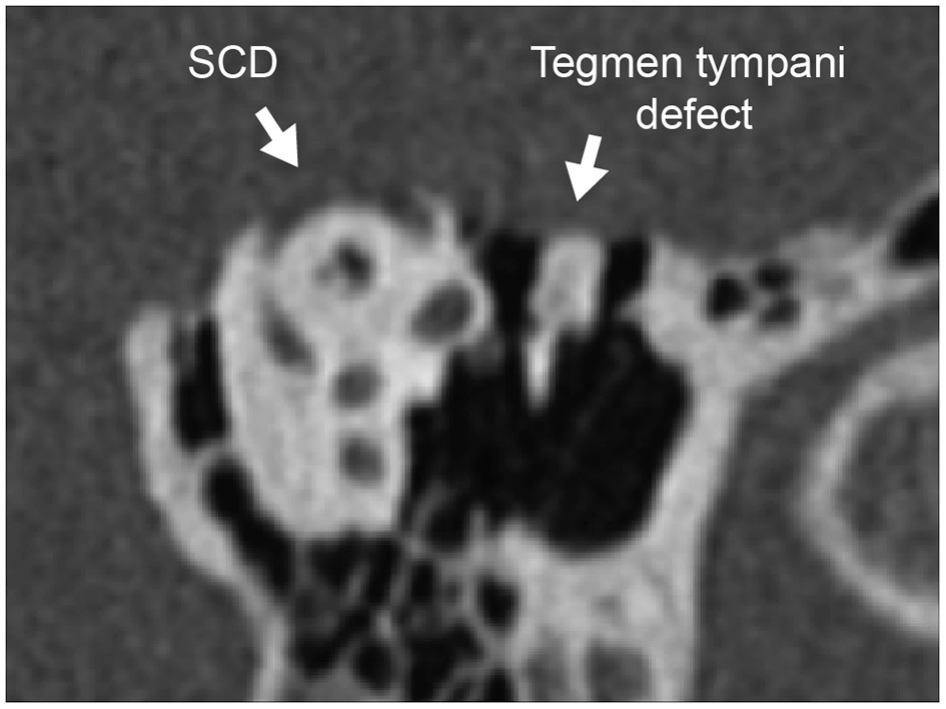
Concomitant tegmen tympani defect with dural herniation. CT of the left ear reformatted to the Pöschl plane demonstrates tegmen tympani bony defect of the skull base and SCD of the arcuate eminence. Herniation of the temporal lobe into the epitympanum can reduce ossicular motion, contributing additionally to the air-bone gap due to SCD and elevating cVEMP threshold (thus masking the lowered cVEMP threshold effect of SCD).
